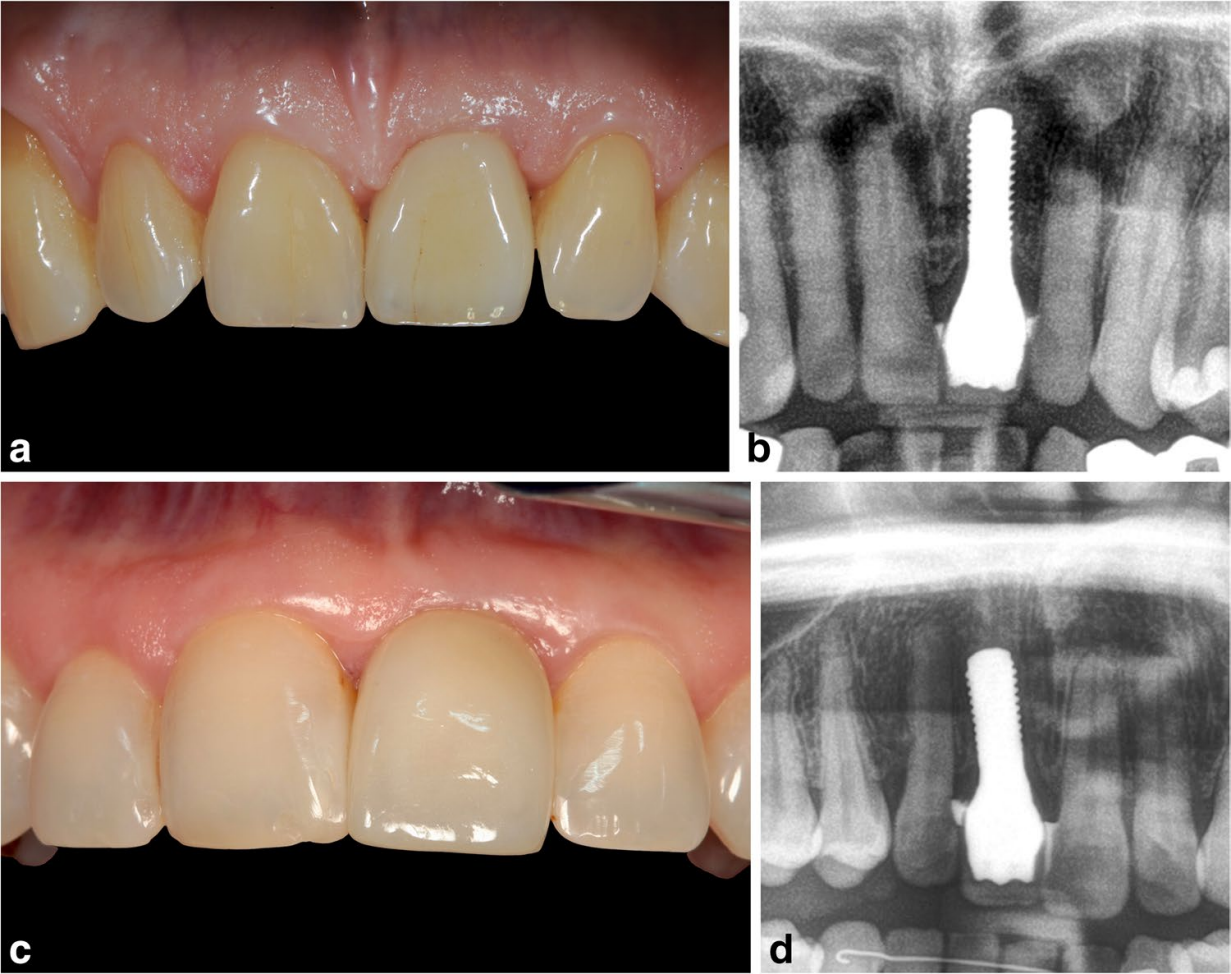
A Clinical follow-up image and B radiographic control of an immediate zirconia implant after 8 years. C Clinical follow-up image and D radiographic control of a delayed-implant case
